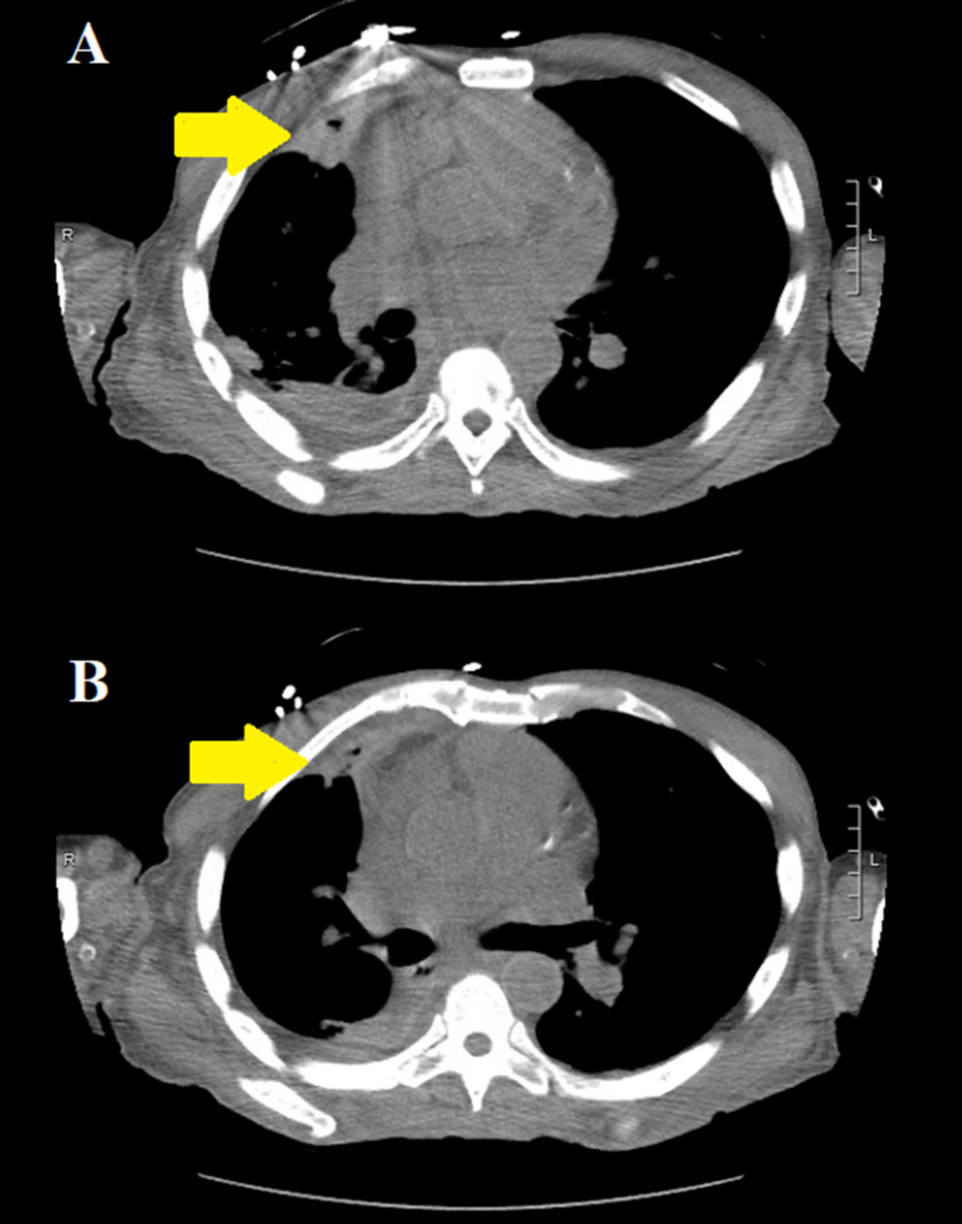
Repeat axial chest CT without intravenous contrast, four days after the second CT scan, demonstrating decreased volume and near complete resolution of gas within the right atrium (yellow arrows in panes A and B).
